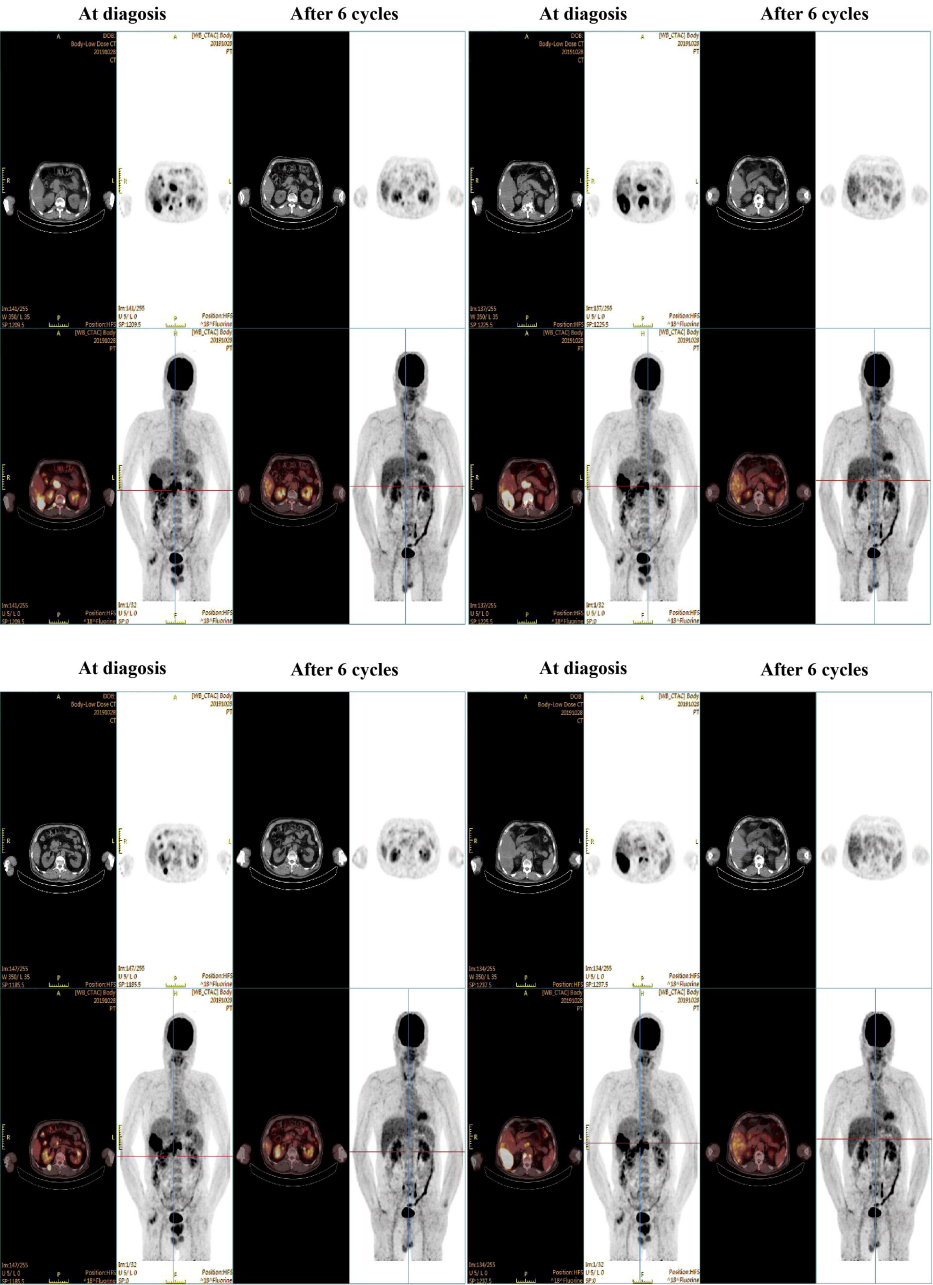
PET-CT scans at diagnosis and after 6 cycles of systemic therapy.
